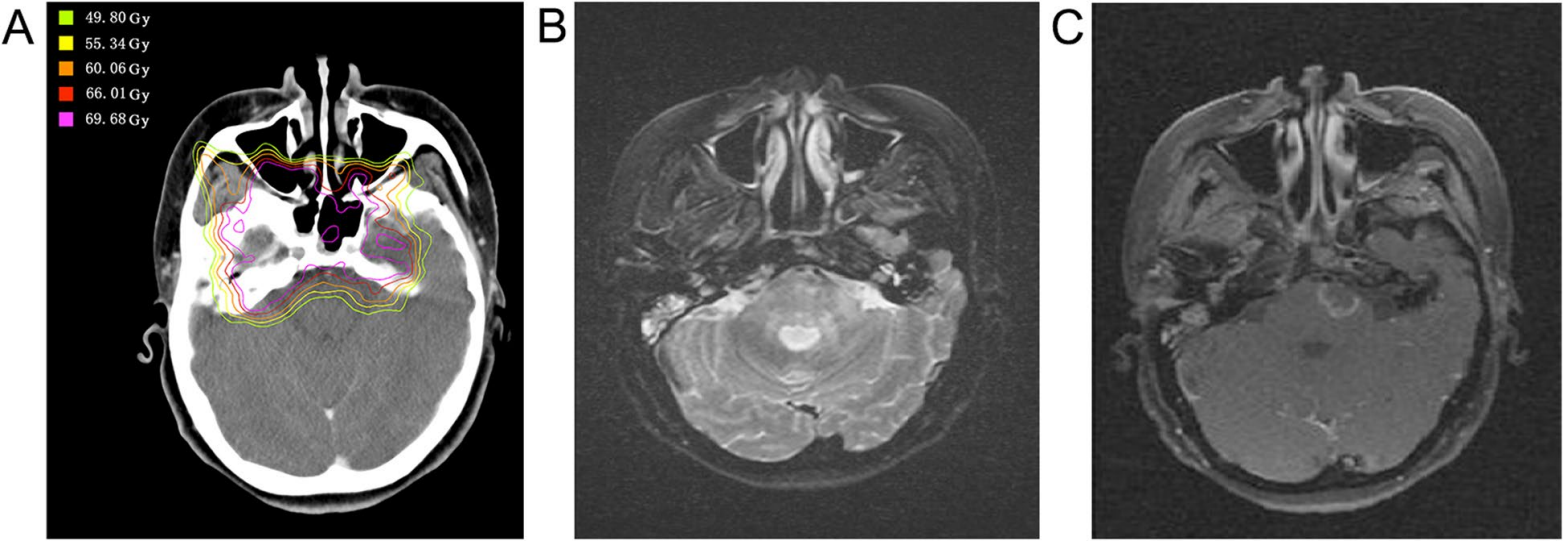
Typical presentation of brainstem necrosis on MRI of a 37-year-old woman, 18 months after IMRT. Significant edema and irregular enhancement in the high-dose area of the left pons was observed. a IMRT dose distribution around the brainstem, in which the max dose was located in the left front of pons; (b) T2-weighted image; and (c) contrast-enhanced T1-weighted image
